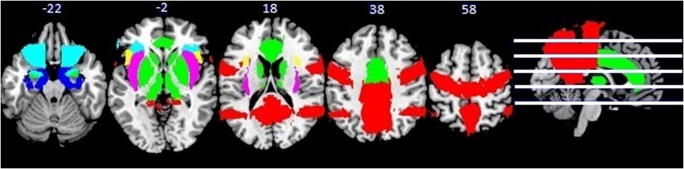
Brain activation patterns (overweight and obese > healthy controls) of taste modalities (bitter, salty, and fat) compiled from studies in Table 1. Red (*fat taste*): BOLD contrast (overweight > healthy controls) to high-fat, high-sugar milkshake in right insula/operculum, precentral gyrus, angular gyrus, bilateral precuneus, posterior cingulate (Bohon et al., 2017). Green (*bitter taste*): BOLD contrast (obese > healthy controls) to quinine-hydrochloride solution in insula, ACC, OFC, amygdala, putamen, pallidum (Szalay et al. 2012). Blue (*salty taste*): [^18^F-FDG] glucose metabolism (overweight and obese > healthy controls) following sodium chloride solution in insula, OFC, parahippocampus (Li et al., 2017). Pink: Overlapping brain activation in insula in response to fat, salty, and bitter tastes. Turquoise: Overlapping brain activation in OFC in response to bitter and salty tastes. Yellow: Overlapping brain activation patterns in response to fat and bitter tastes the numbers above the brain slices indicate the Z-coordinate in MNI space
